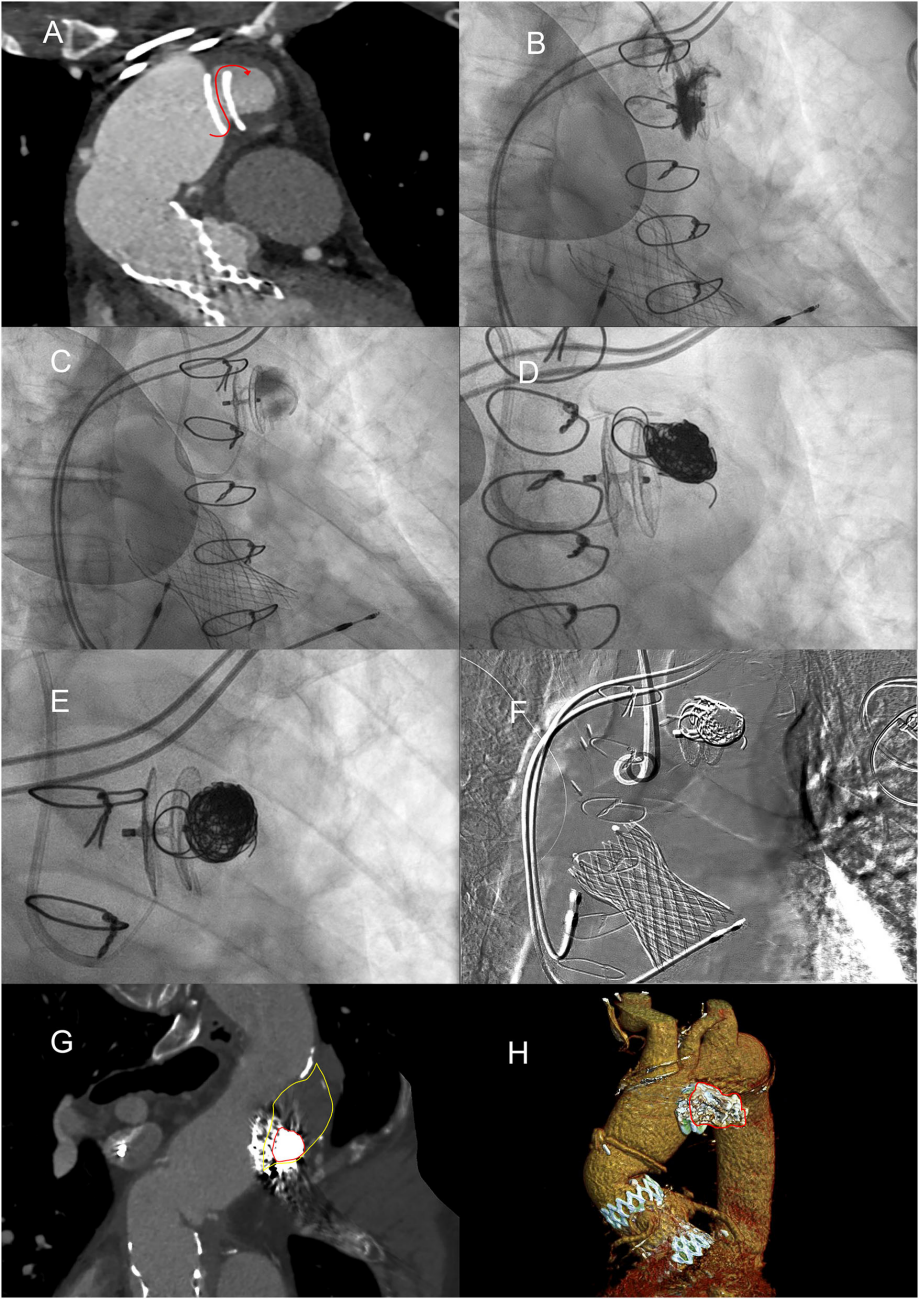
Embolization of the residual pseudoaneurysm, in volumetric expansion. (A) MPR reconstruction demonstrates continuity between the aortic lumen and the pseudoaneurysm lumen with flow direction highlighted by the red curved arrow. (B) Found the direction of blood flow with selective angiography, after performing CT and during transesophageal ultrasound. (C) Angiography demonstrating the opacification with microcatheter of the residual pseudoaneurysm sac. (D) Controlled release of coils by means of a microcatheter. (E) AAP completely filled with platinum embolization coils, enlaced with poly-lactide-co-glycolide acid (PGLA) fibers. (F) Digital subtraction angiography showing all “metallic” devices. (G) MPR curved reconstruction from angio-CT after placement of coils in the lumen of the AAP. (H) Angio-CT VR reconstructions of the thrombosed pseudoaneurysm with coils.
